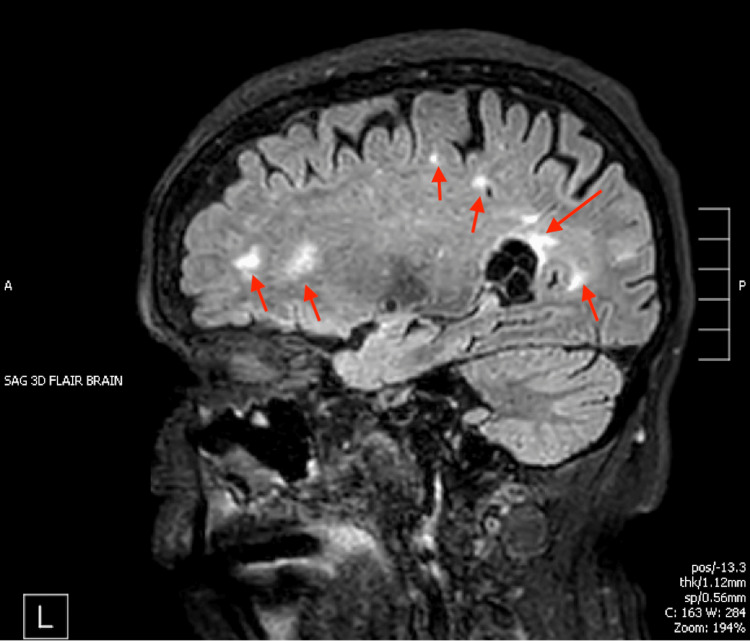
Sagittal FLAIR image demonstrating bilateral subcortical hyperintense signal consistent with vasogenic edema (ARIA-E) ARIA-E, amyloid-related imaging abnormalities with vasogenic edema; FLAIR, fluid-attenuated inversion recovery
